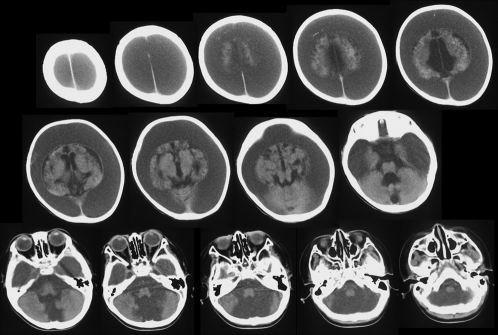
Brain computed tomography of a 6-year-old boy (indicated with * in Table 1). He suffered from severe cortical atrophy and massive subdural fluid collection.
